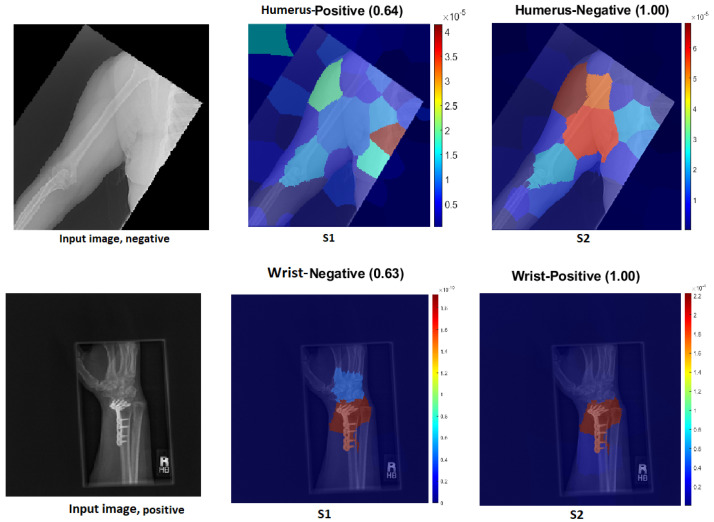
LIME and score LIME for the humerus and wrist X-ray images. The correct classification is negative for the humerus and positive for the wrist.
